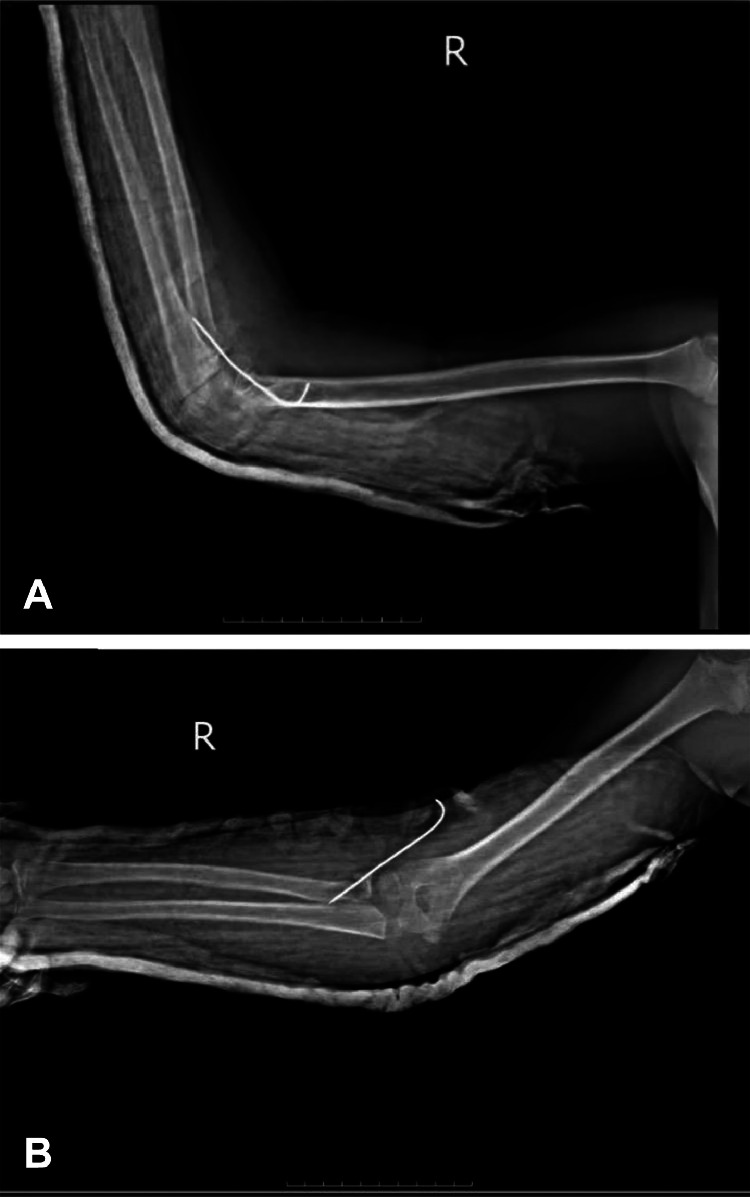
A: Post-operative lateral radiograph of the right elbow Plain radiography (X-ray) in lateral view with the elbow flexed at 90°, showing reduced elbow joint with K-wire fixation of the radial head (arrow). Note the restoration of normal articulation between the distal humerus and the proximal radius and ulna. B: Post-operative anteroposterior radiograph of the right elbow Plain radiography (X-ray) in anteroposterior view with the elbow partially extended, demonstrating appropriate K-wire placement through the radial head (arrow) and anatomic reduction of the joint.
